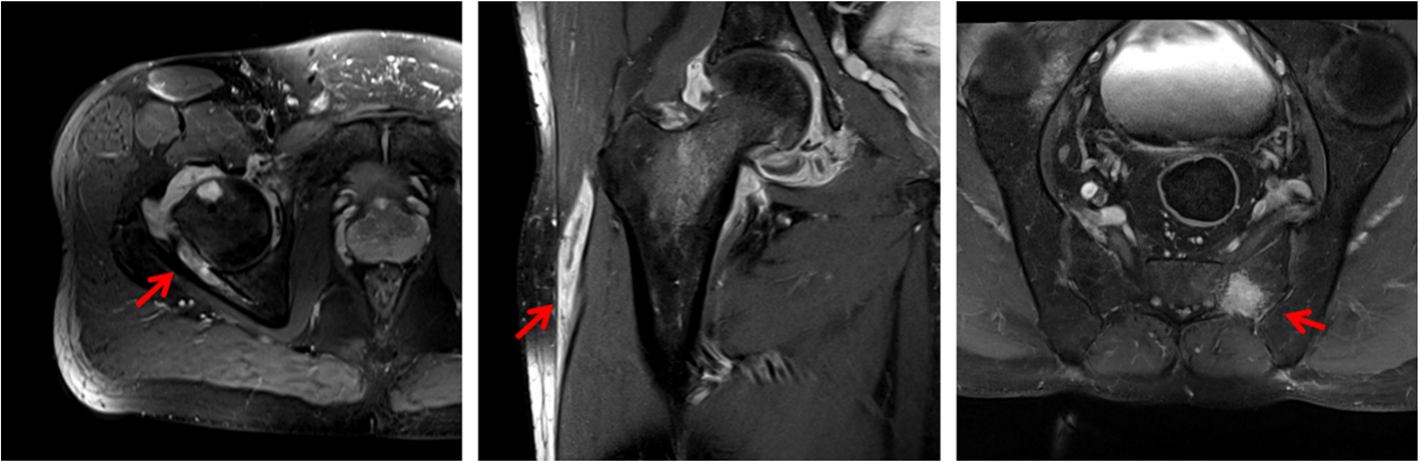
Magnetic resonance imaging scans; T2-weighted images. A right-sided coxitis (*right*) with a trochanteric bursitis (*middle picture*) as well as a left-sided osteitis of the sacral bone (*left*) are indicated with *red arrows*
